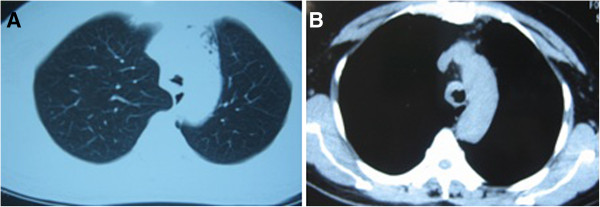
Computed tomography (CT) scan of the chest showed multiple soft tissue masses adjacent to the trachea, causing diffuse narrowing of the tracheal lumen. (A) pulmonary window. (B) mediastinal window.
